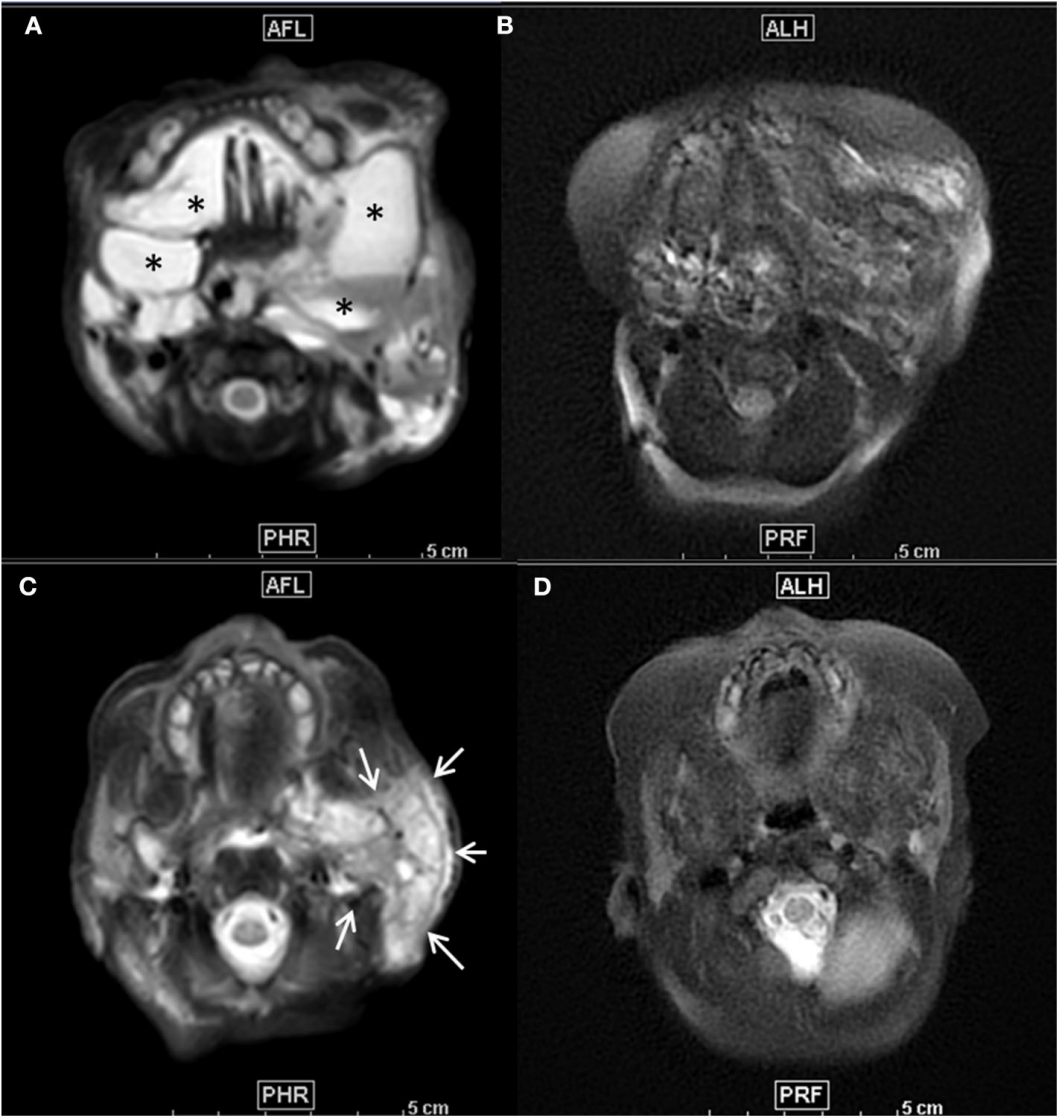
(A–D) Axial T2-weighted fat suppressed MR images before treatment (A,C) showed mixed macro- and microcystic extensive cervico-facial lymphatic malformation with diffuse involvement of the sublingual space and both cheeks by large macrocysts (*) (A) and extensive infiltration of the left parotid space by a large microcystic component, as demonstrated by the diffuse T2 hyperintensity of the whole left parotic space (arrows) (C). Seven months after initiation of the Sirolimus and after 3 percutaneous procedures of sclerotherapy, follow-up axial T2 weighted images at the same anatomical levels (B,D) demonstrated a major decrease of both macrocysts (B) and microcystic infiltration as demonstrated by the important decrease of T2 intensity of the parotid space (D).
